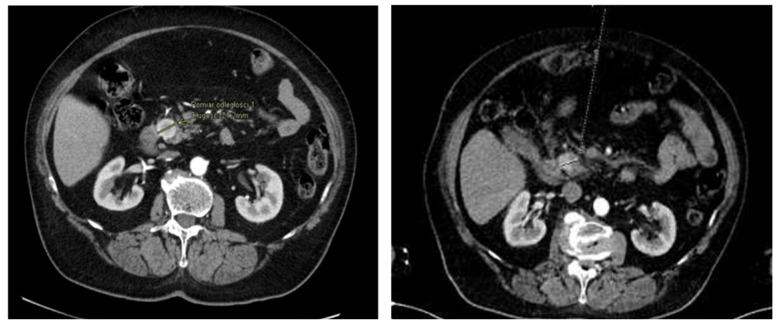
Second case. Comparison of CT performed in June 2016 (left image) and June 2017 (right image): stable tumor dimensions with a decrease in the level of contrast enhancement in the left half of the pancreatic tumor after SBRT.
